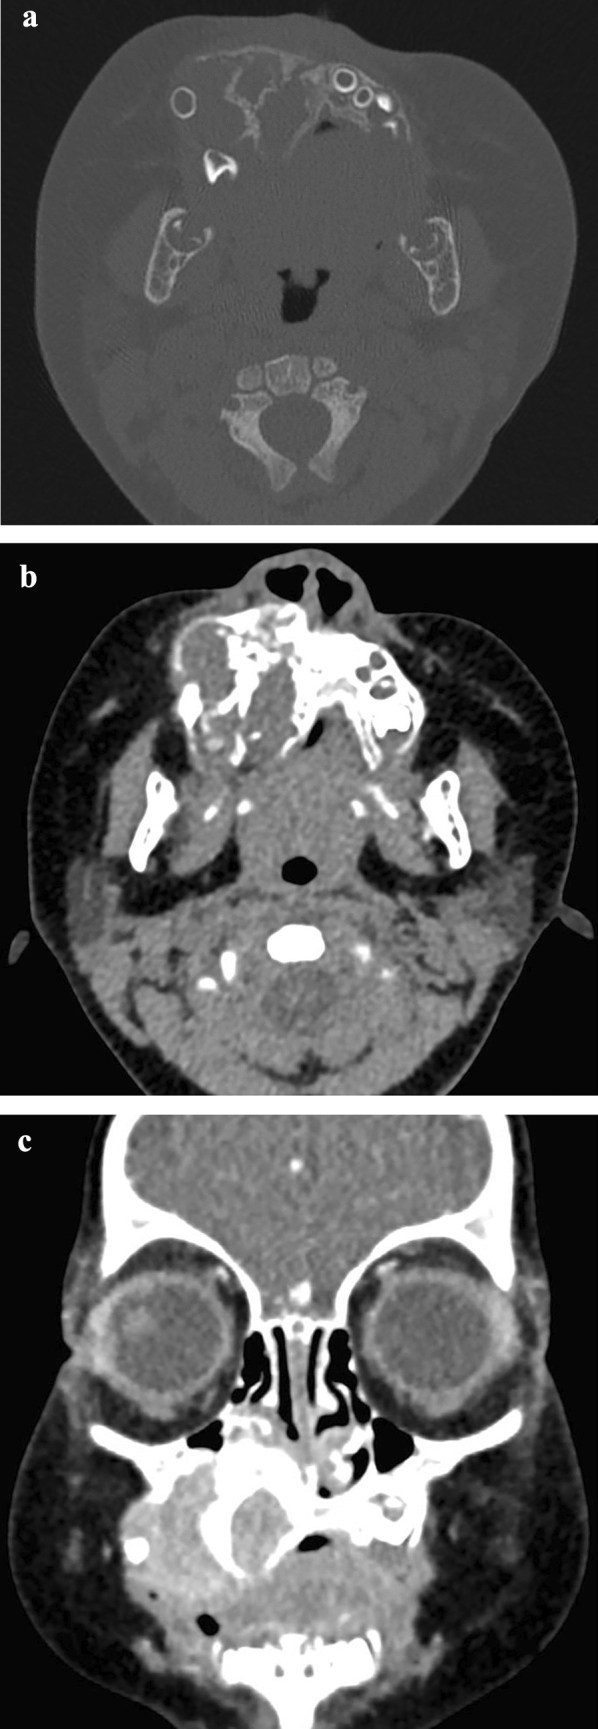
Case 1. a/b axial CT images demonstrated a multi-locular soft-tissue mass occupying the right maxillary sinus and leading to irregular bony destruction. Cortex expanded and lost continuity without periosteal or bony proliferation. Displaced tooth germ was noticed. c coronal contrast-enhanced CT showed heterogeneous enhancement of the lesion
